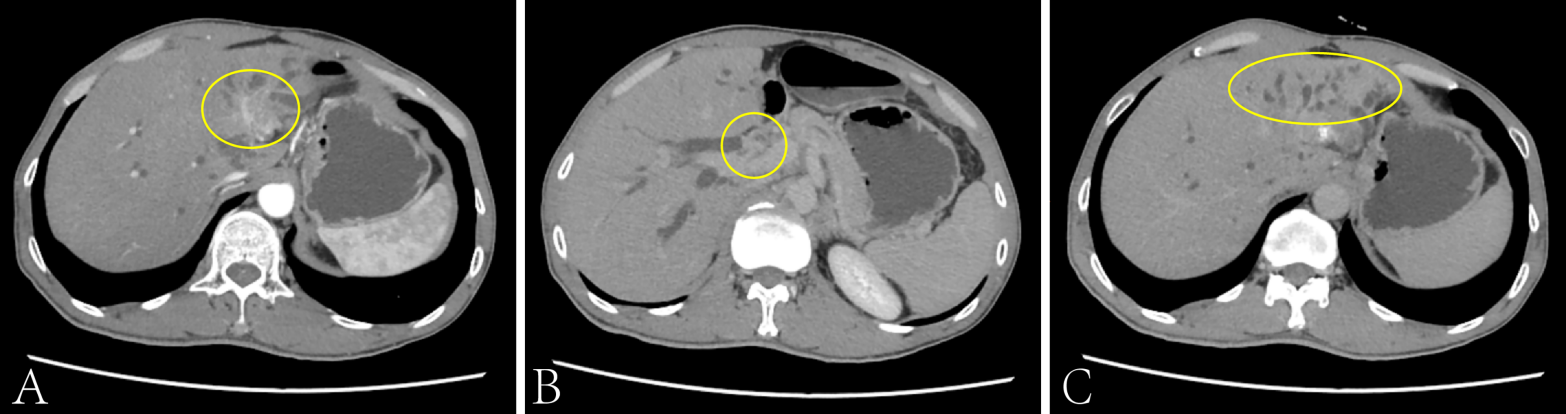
The CT scan of the patient’s liver on Dec. 2020. The main metastatic lesion (3.4 × 2.6cm) in the left and caudate lobe of the liver (A), the metastatic nodule (1.5 × 1.1cm) in the hepatic portal (B) and the localized common bile duct compression (C).
